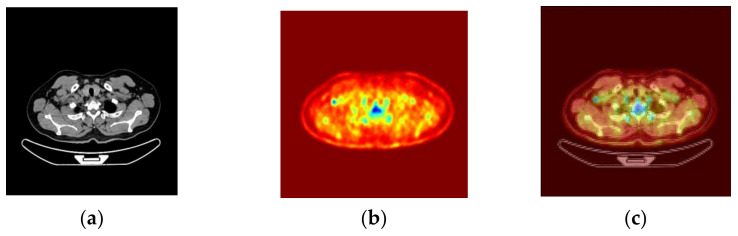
Fusion of PET and CT. (a) CT image. (b) PET pseudo-color image with 256 colors. (c) The PET image is fused with the CT image.
